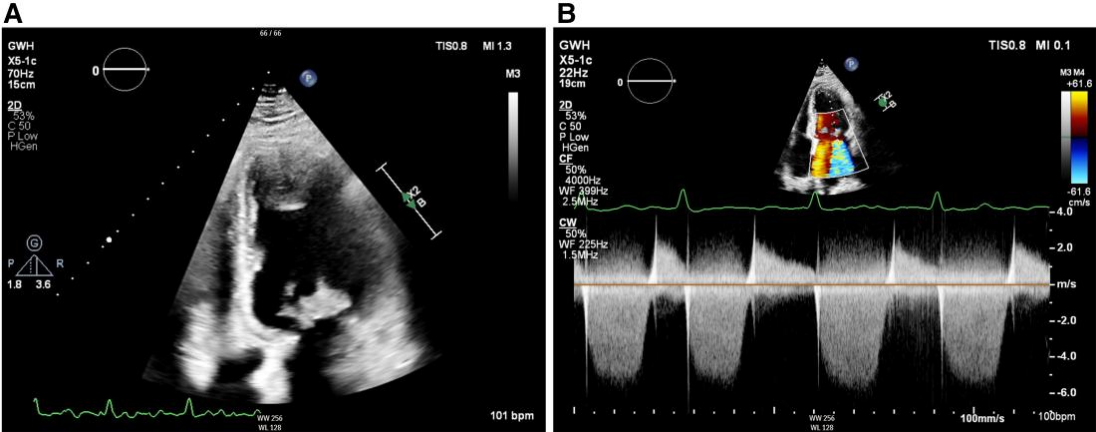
Transthoracic echocardiogram showing (*A*) a large mass associated with the mitral valve in apical four-chamber view; (*B*) continuous wave Doppler suggestive of severe mitral regurgitation.
